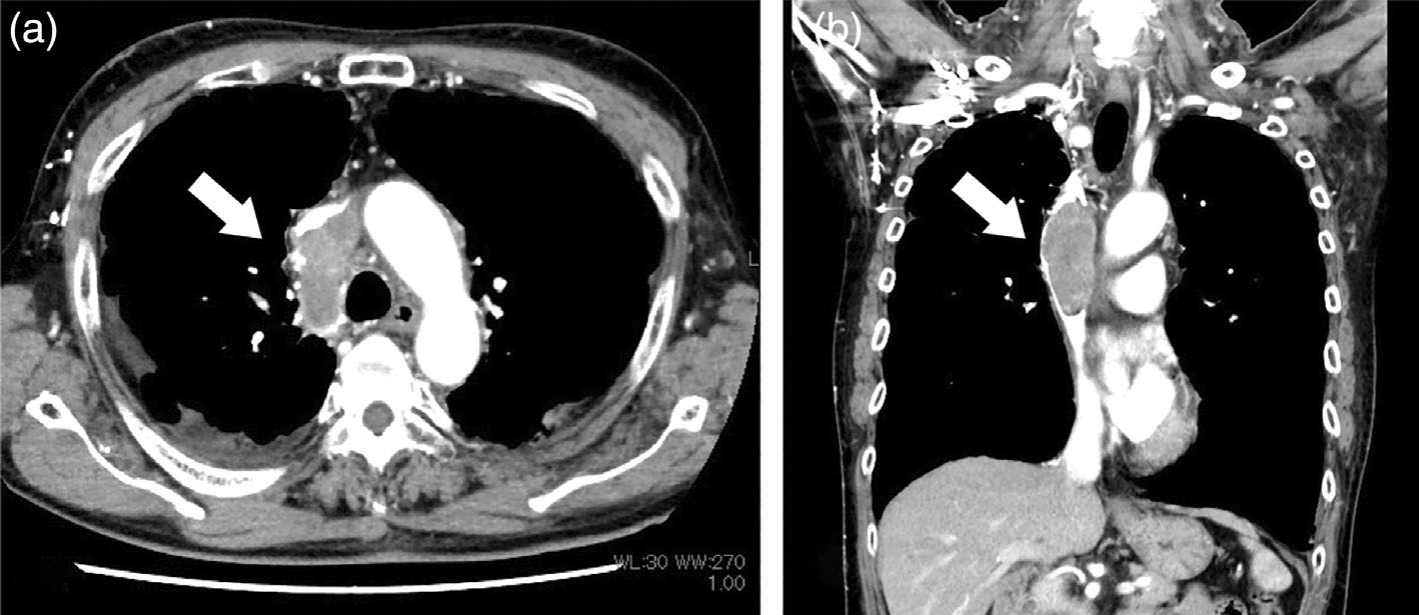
Axial (a) and coronal (b) enhanced chest computed tomography images showing a mass (arrow in each panel) in the azygos vein and the superior vena cava.
